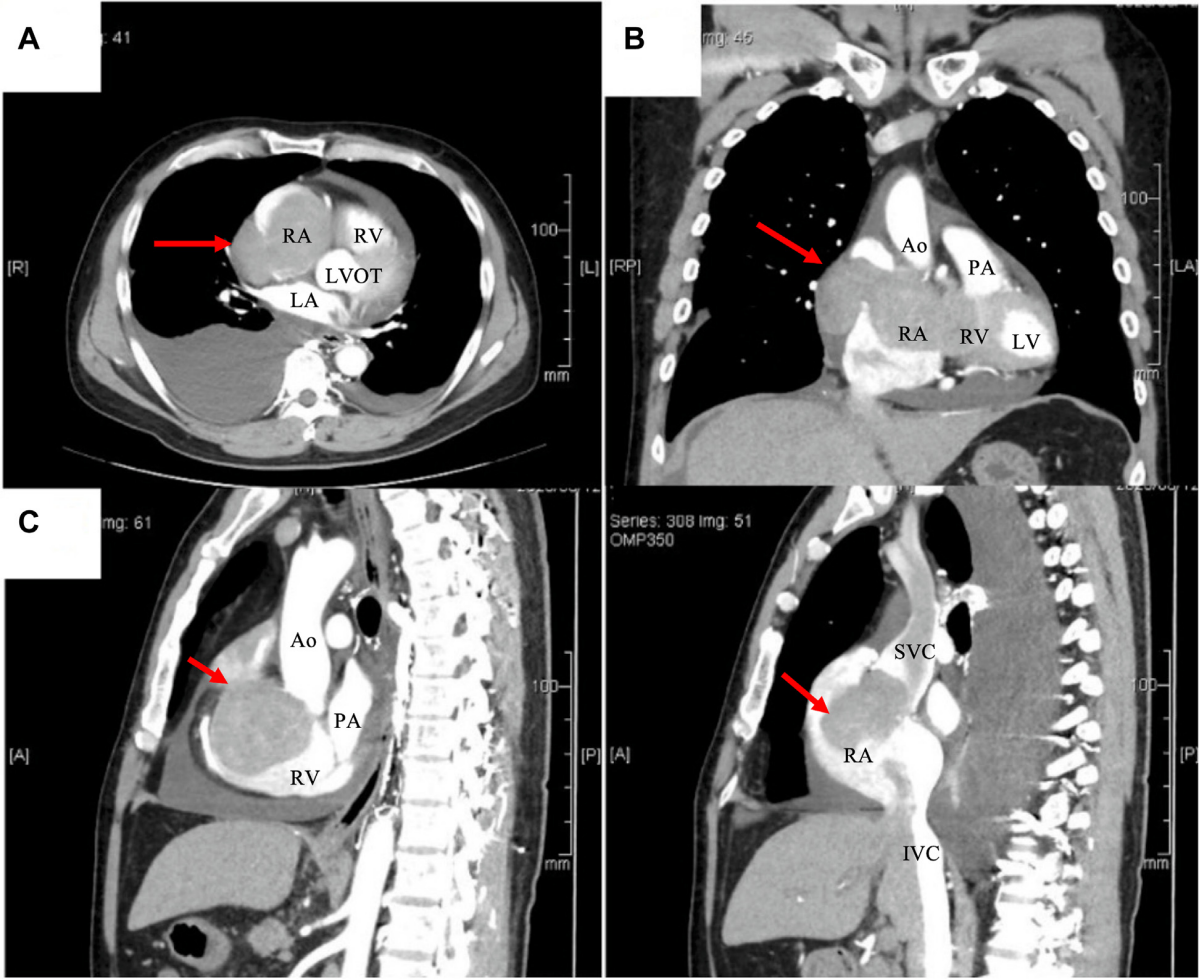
Enhanced Cardiac Computed Tomography (A) Axial view; (B) coronal view; (C) sagittal view. Enhanced computed tomography shows a 9.8 × 6.0 cm tumor mass (red arrows) and a large pericardial and pleural effusion. The mass extends outside of the heart, breaking though the right atrium free wall. It is smoothly marginated and isodense. Ao = aorta; IVC = inferior vena cava; LA = left atrium; LV = left ventricle; LVOT = left ventricular outflow tract; PA = pulmonary artery; RA = right atrium; RV = right ventricle; SVC = superior vena cava.
